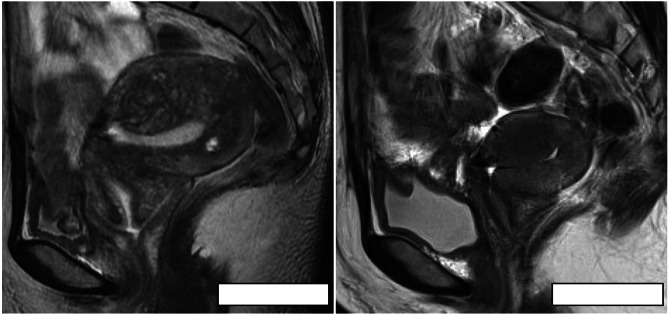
Representative uterine images of T2-weighted MRI before and after pregnancy in a patient with adenomyosis. The left and right images represent the status of adenomyosis before and after pregnancy, respectively. Scale bar = 5 cm. T2WI, T2-weighted image
